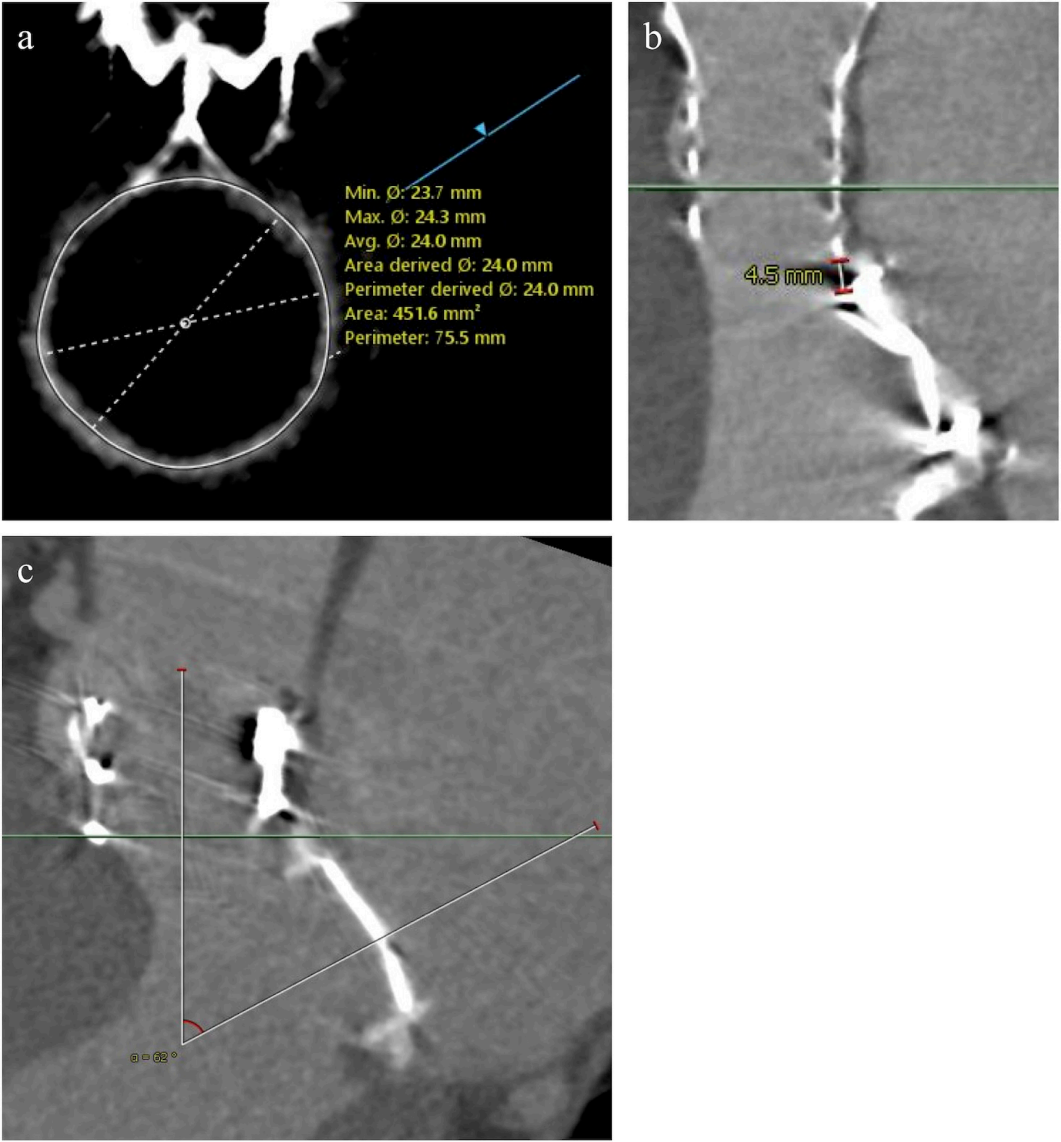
Postprocedural MDCT measurement. (a) Size of the mitral prosthesis housing. (b) The distance between the newly implanted THV and mitral prosthesis housing. (c) The angle between the mitral prosthesis and newly implanted THV. LVOT = left ventricular outflow tract; MDCT = multidetector computed tomography; THV = transcatheter heart valve.
